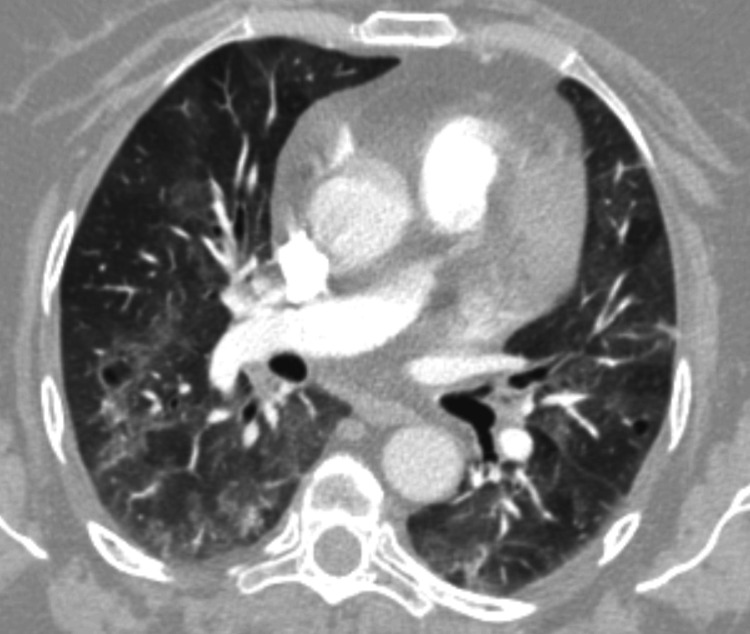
CT pulmonary angiogram demonstrates diffuse bilateral ground-glass changes with some mosaic changes, suggestive of pneumonitis.
